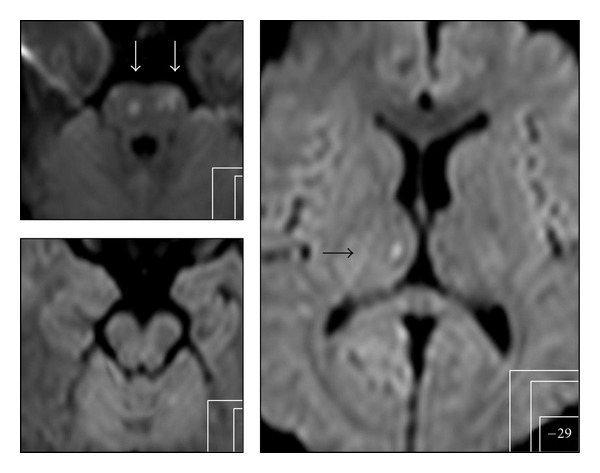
Very few DWI lesions in follow-up MRI 24 h after symptom onset in pons (white arrows) and in the right thalamus (black arrow) and no neurological deficit.
